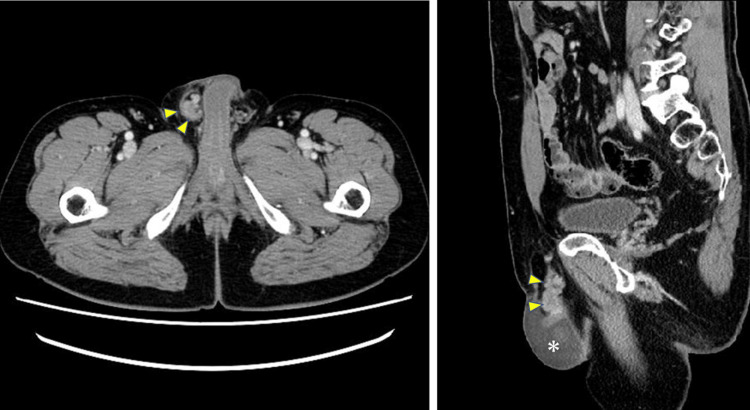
Abdominal CT scan. Left image: a tumor shadow is observed within the right swollen spermatic cord (arrows). Right image: a tumor shadow is observed within the right spermatic cord extending from the right inguinal canal to the epididymal region (arrows) with dilated processus vaginalis peritonei. A hydrocele is observed in the right scrotum (asterisk).
